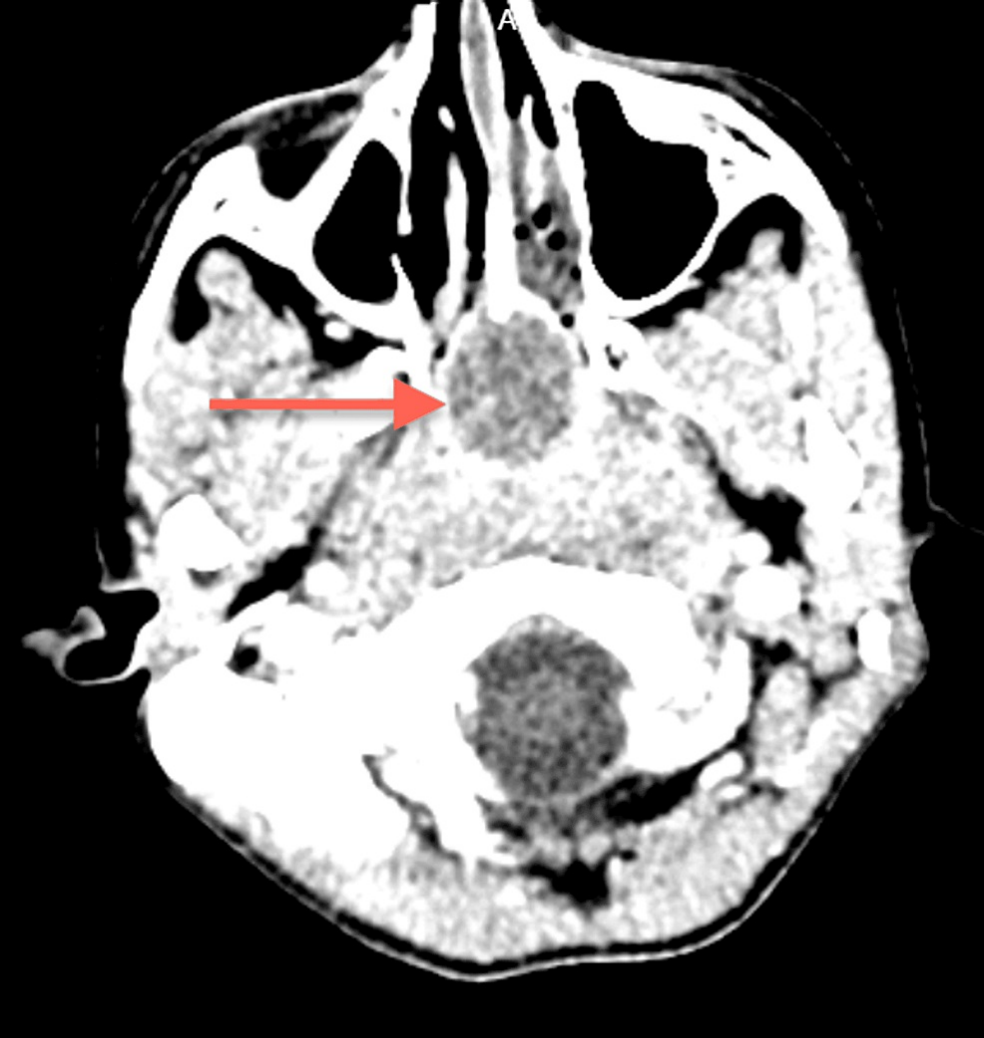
CT Neck CT of the neck showing the adenoid region with a 4.6x2.7 mm cystic lesion remodelling the floor of the sphenoid sinus, displacing the uvula anteriorly and compromising the nasopharyngeal airspace.
